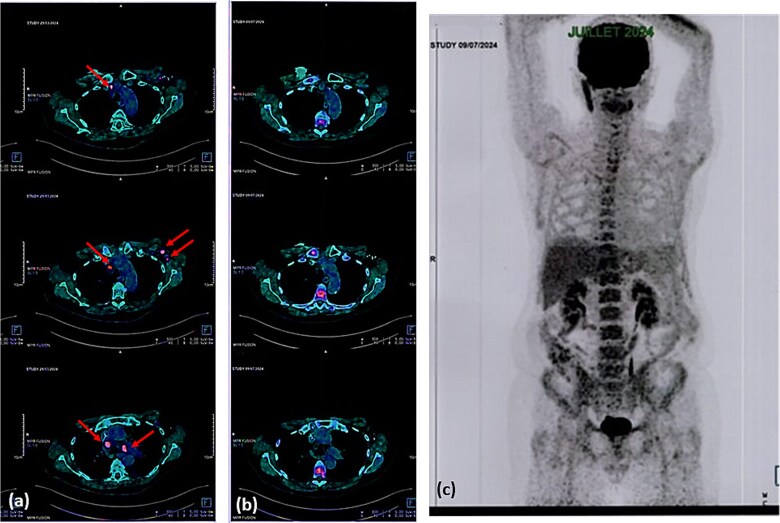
Comparative FDG PET/CT study between march 2024 (a) and July 2024 (b) showing complete metabolic regression of the hypermetabolic foci in the mediastinal and left axillary lymph nodes (arrows). MIP view from July 2024 (c). FDG: Fluorodeoxyglucose, PET/CT: Positron emission tomography/computed tomography, MIP: Maximum intensity projection.
